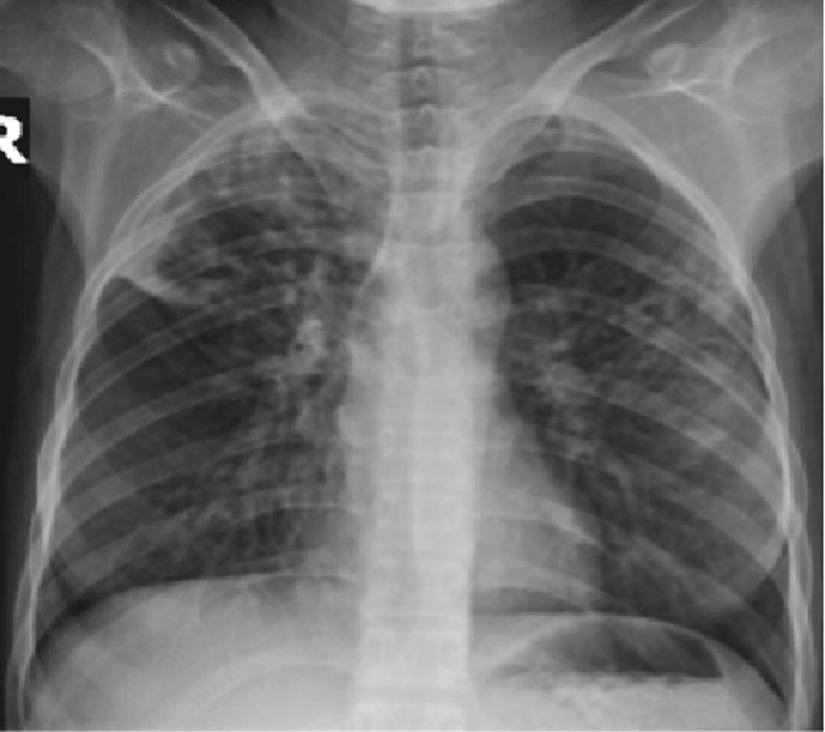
Chest radiograph showing bilateral upper and mid-lung infiltrates due to MDR-TB in a patient suffering from SLE.
